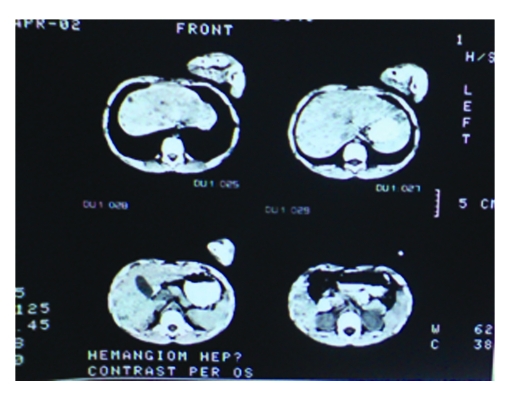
Computed tomography showing multiple liver low–density lesions
